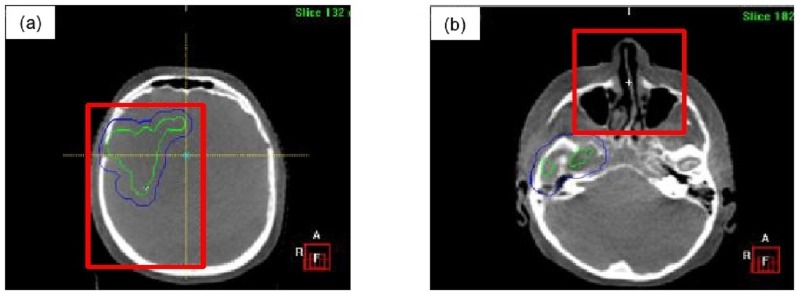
Example regions of interest (red outline) for automatic CBCT image registration. Representative coronal images for: a) target, b) nose.
